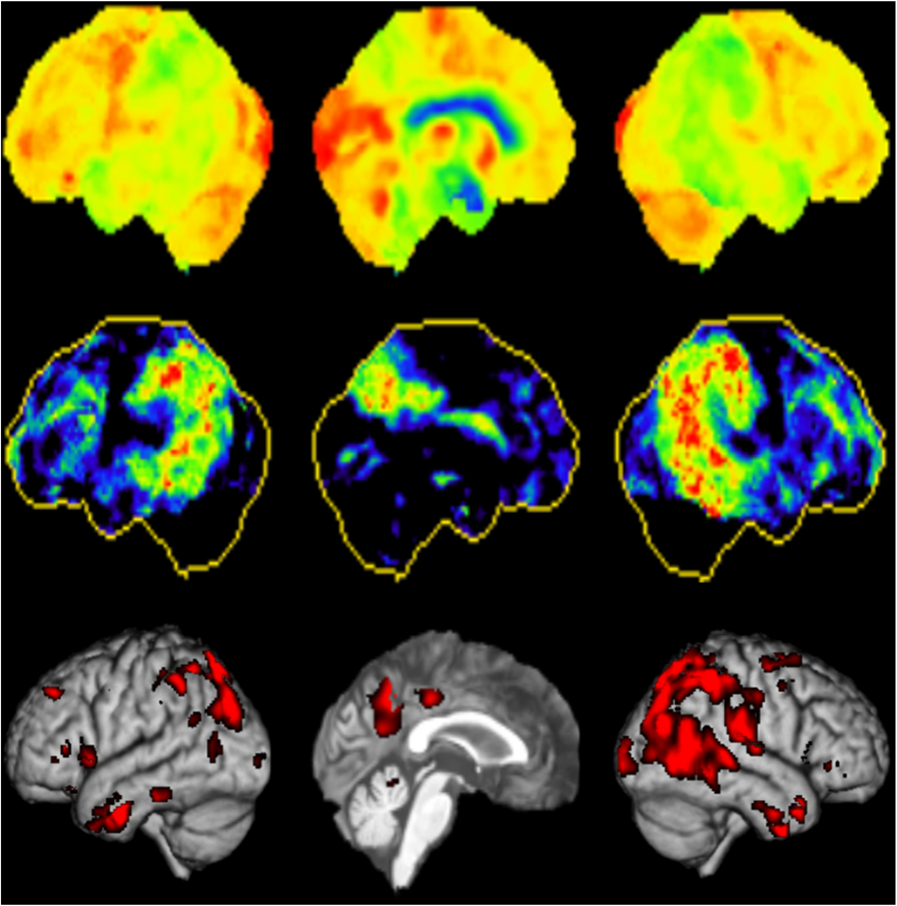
Positron emission tomography of the brain with F-18-labeled fluorodeoxyglucose (FDG-PET) and three-dimensional surface representation of brain regions with grey matter reduction of our 59-year-old AD patient compared to 18 healthy controls. Top: regional uptake of radiolabelled glucose; the left lateral, left medial and right lateral views of the brain are presented. Middle: regional metabolic reduction compared to age-matched healthy controls according to the methodology of Minoshima et al. [53]. There is an asymmetric metabolic reduction parietotemporal and in posterior cingulate cortex with a more pronounced reduction of glucose metabolism in the right hemisphere. Bottom: color-coded brain matter reduction. Z-values of grey matter reduction are projected on T1-weighted, averaged brain surface of healthy control subjects
